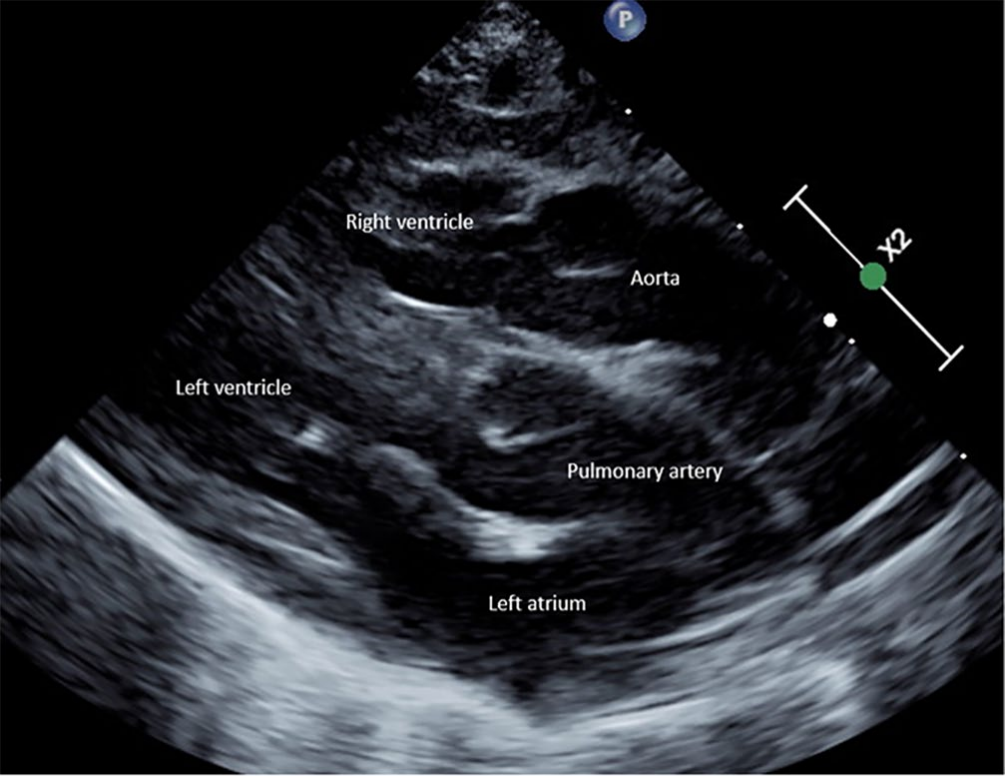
Parasternal long‐axis view on transthoracic echocardiogram in our patient demonstrating D‐transposition of the great arteries. The aorta is anterior and rightward relative to the pulmonary artery and arises from the right ventricle
